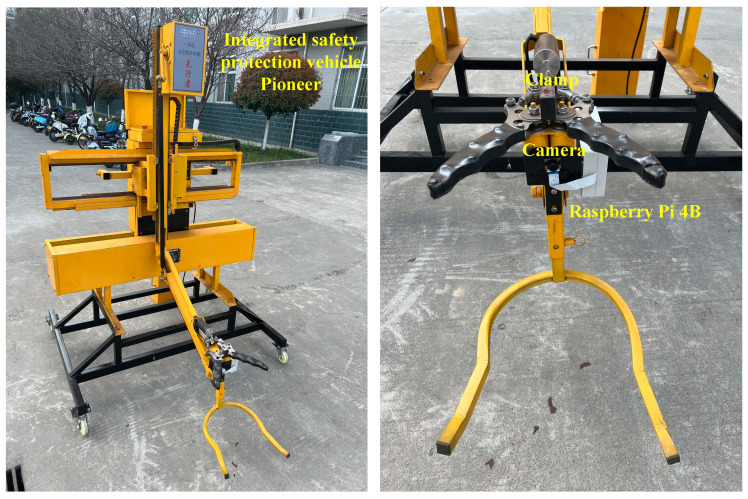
The experimental platform of automatic traffic cone retractor.As shown in Figure 13, when the operation mode is traffic cone retraction, the four-link mechanism’s motor lowers the manipulator to the retraction height. Once the cone enters the clamping range, the ultrasonic sensor in front of the manipulator feeds an electrical signal back to the PLC, and the machine vision equipment starts tracking and detecting. It transmits the traffic cone coordinates and contour information to the PLC. Subsequently, the PLC drives the four-link mechanism, gear rack slide rail, and electronic push-pull rod to move, adjusting the width of the manipulator’s extension and the vertical position, as well as the angle of the clamp. Finally, the clamp grips the cone, and the manipulator lifts the cone to the upper limit. After the infrared sensor above the clamp detects a human hand, the clamp releases the cone. The operator can then remove the cone, and the manipulator moves to the lower limit for the next recovery operation.
